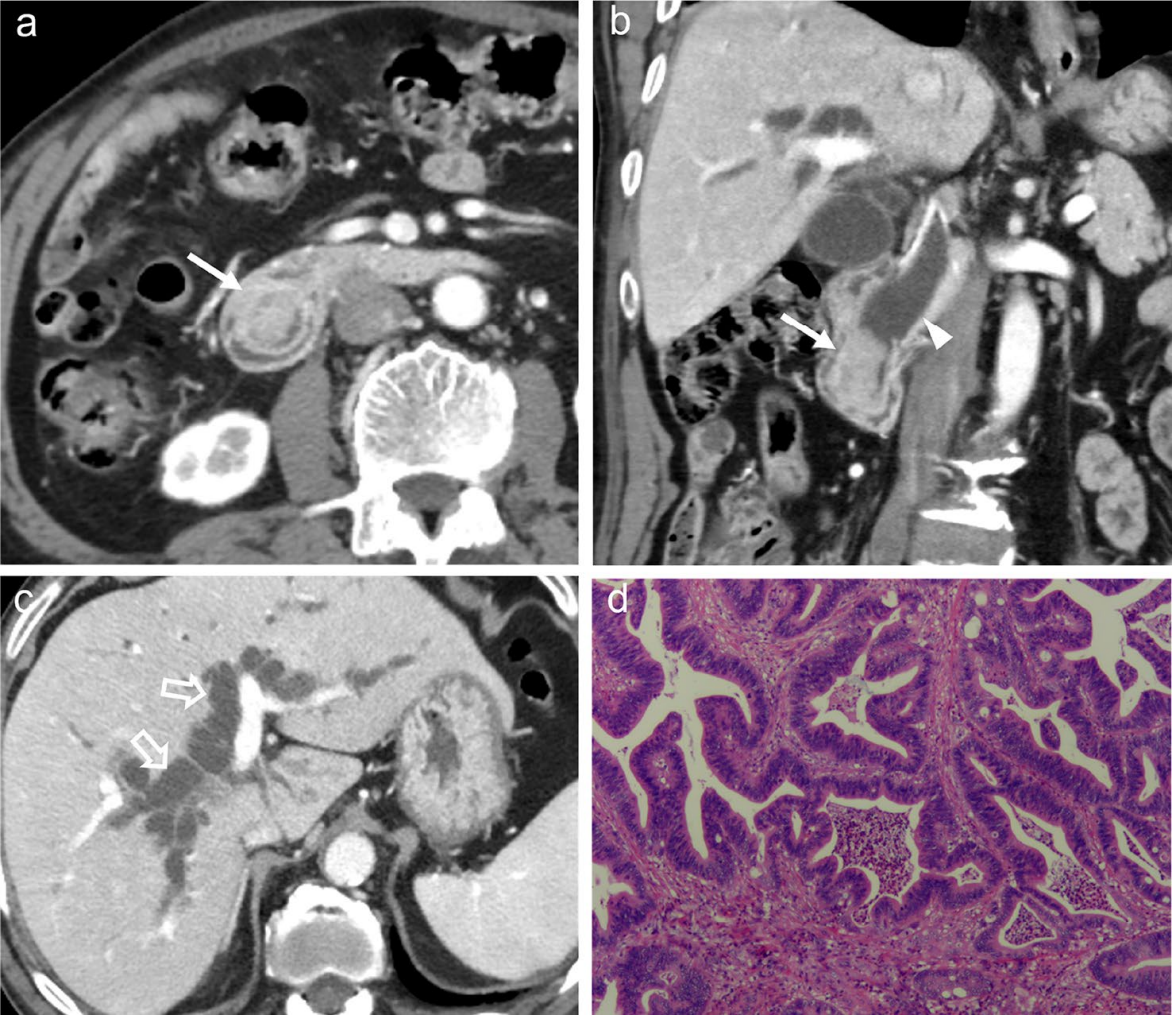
An example from a 72-year-old man who complained of epigastric pain and skin pruritus. Axial (a, c) and coronal reconstructed images (b) of enhanced CT in portal venous phase show a periampullary mass with enhancement degree similar to that of the duodenal wall (arrows), with an intrinsic growth pattern. The shape of distal CBD margin is smooth (arrowheads) accompanied by corresponding upstream bile duct dilatation (open arrow). The postoperative pathologic diagnosis of this patient was an intestinal-type periampullary adenocarcinoma (d)
